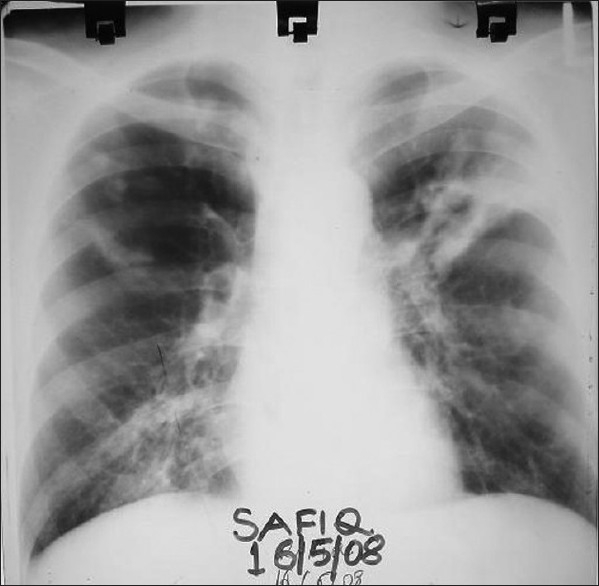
Chest X-ray reveals a large cavity with a mass filled opacity confined to left upper zone and fibrotic changes in right upper zone
